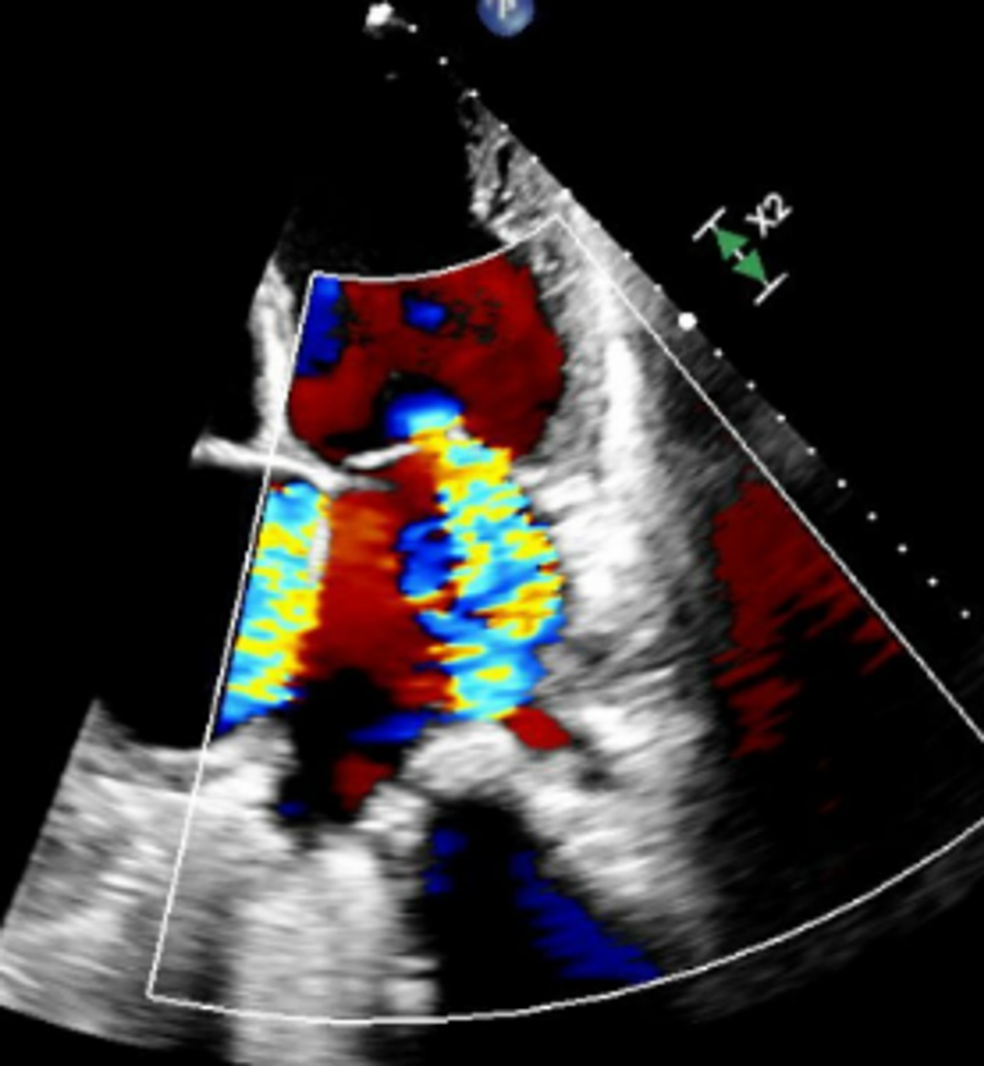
Mitral Regurgitation (four-chamber view on echocardiogram)
